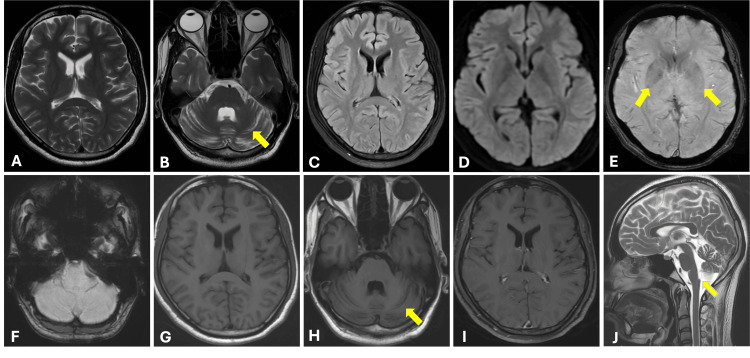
Axial T2-weighted images (A, B) and fluid-attenuated inversion recovery (FLAIR) image (C) show no abnormal signal intensity in the basal ganglia (A, C), with associated cerebellar atrophy (arrow in B). Axial diffusion-weighted imaging (DWI) image (D) demonstrates no restricted diffusion. Axial susceptibility-weighted imaging (SWI) images (E, F) show mild blooming in the bilateral lentiform nuclei (arrows in E) with no blooming foci in the cerebellum (F). Axial T1-weighted images (G, H) reveal normally appearing basal ganglia with mild cerebellar atrophy (arrow in H). Axial post-contrast T1-weighted image (I) shows no abnormal enhancement. Sagittal T2-weighted image (J) demonstrates clival hypertrophy (arrow).
